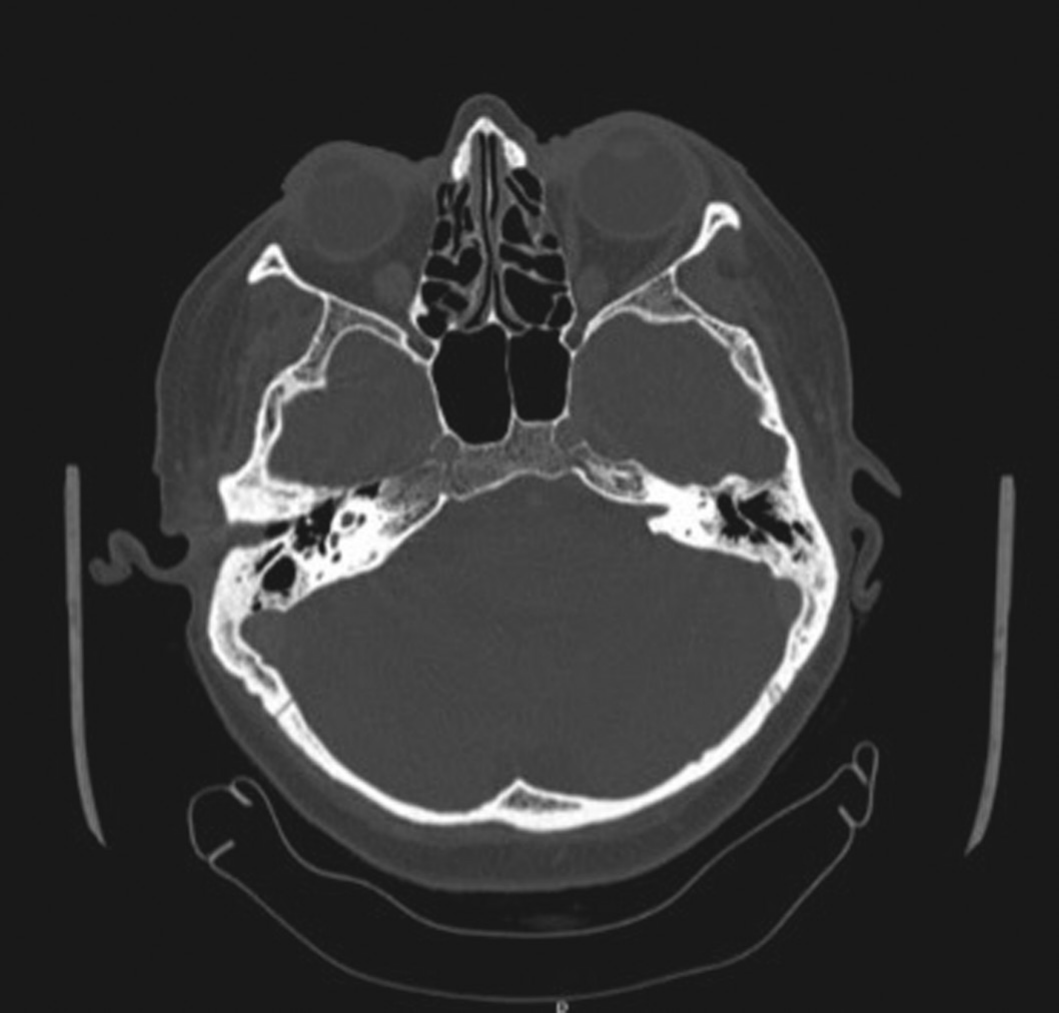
Brain CT scan performed in emergency room, no evidence of acute otitis, mastoiditis, or acute cerebrovascular disease.
